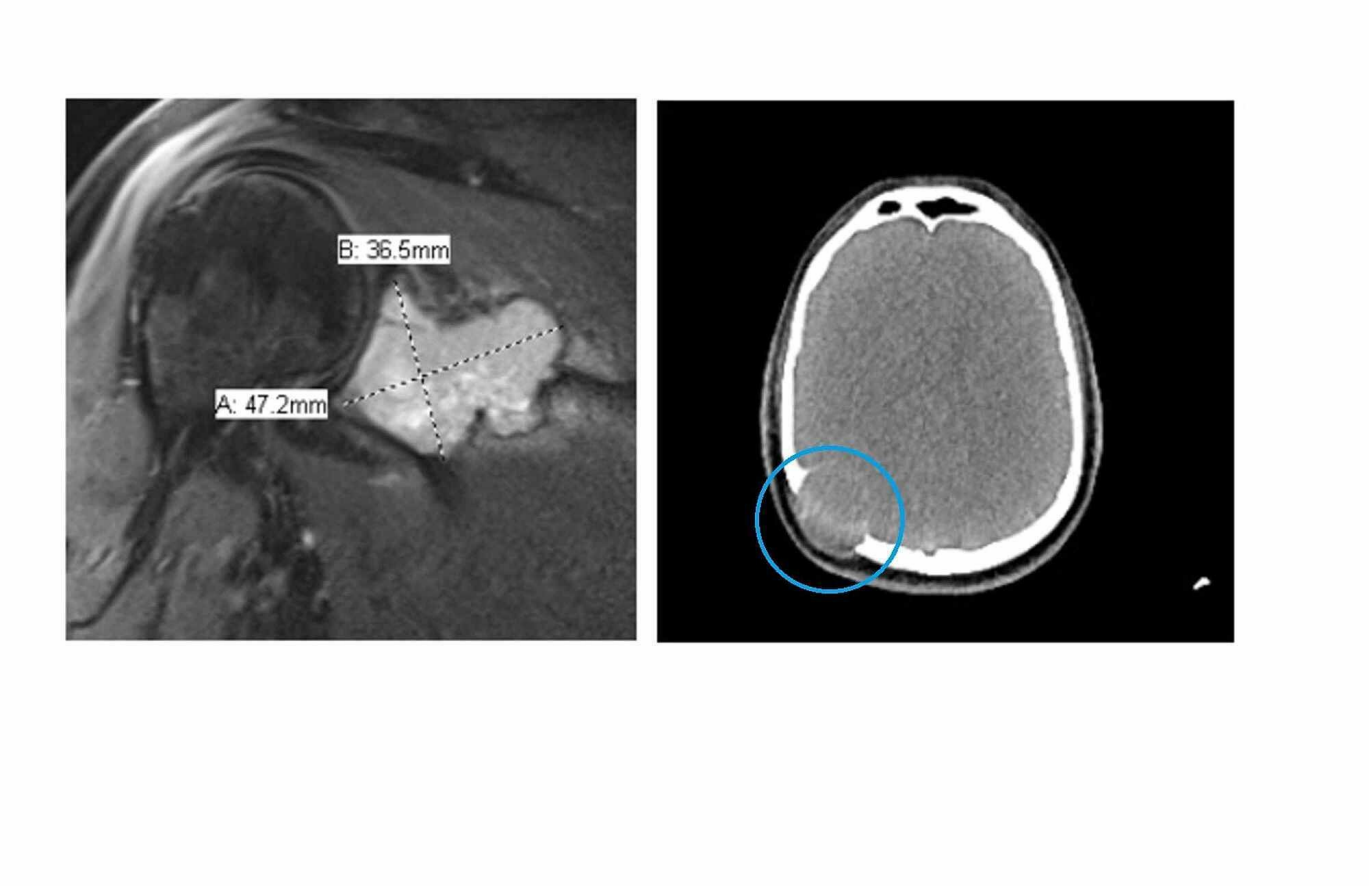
MRI shoulder shows lytic lesion. CT-Brain shows parieto-occipital expansible lesion (blue circle).
